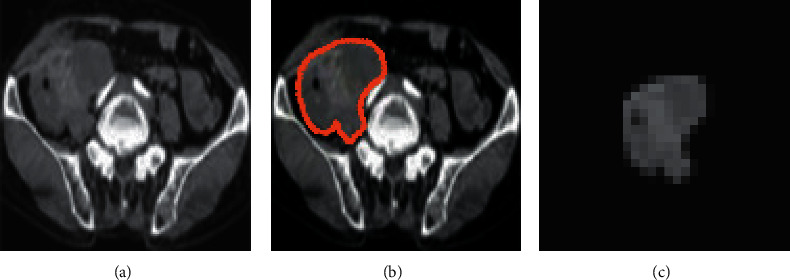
CT image analysis and ROI delineation of primary lesions in patients with colorectal cancer. Case 1, male, 56 years old, CRLM. (a) CT image of a patient with colorectal cancer; (b) lesion manually drawn by the patient; (c) ROI of the drawn lesion.
